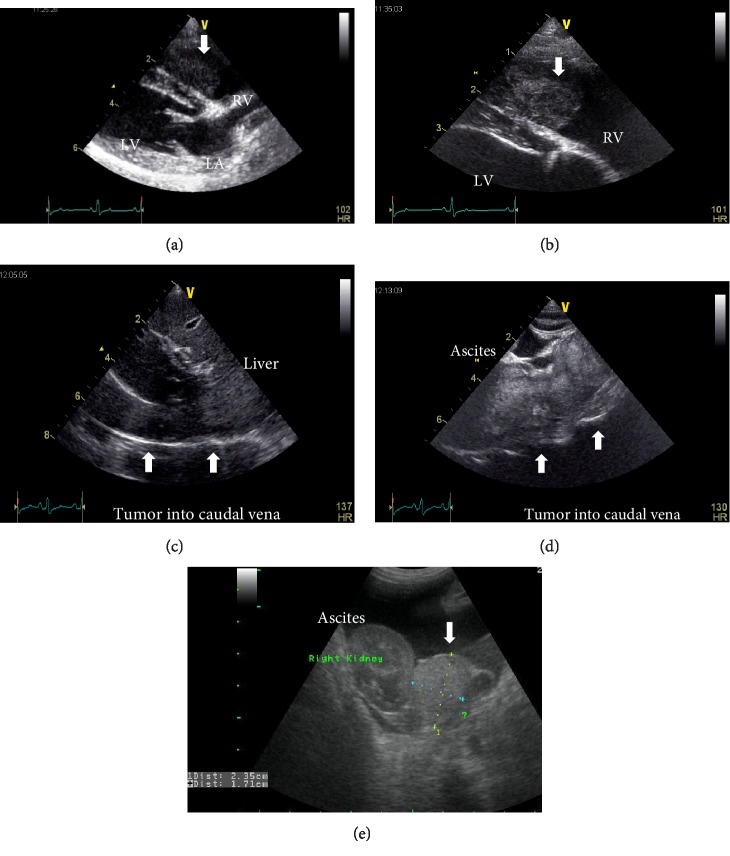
Transthoracic echocardiographs showing tumor invading into the right ventricular cavity on the right parasternal long axis four-chamber view (a). Tumor (arrow) can be seen within the right ventricle. Abdominal echocardiographs showing the tumor (arrow) invading into the caudal vena cava (b), (c), and the tumor and right kidney (d).
